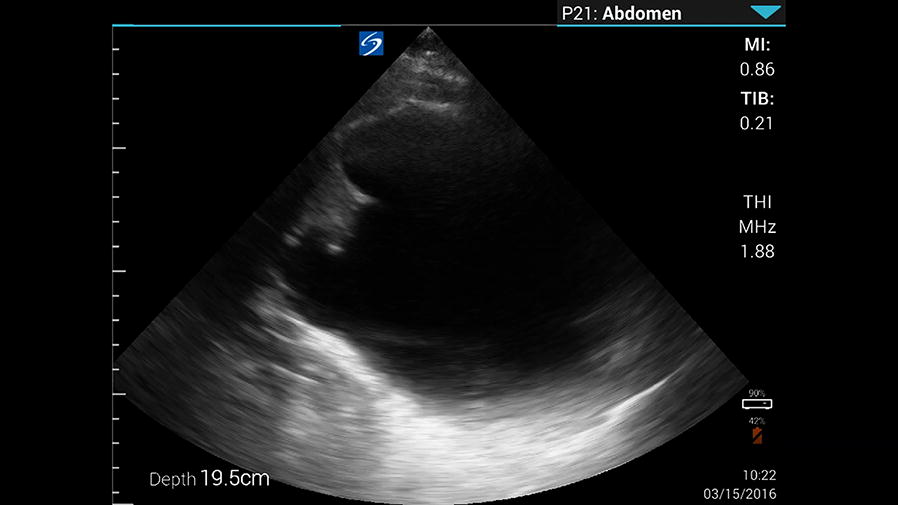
Ultrasound of the patient’s right upper quadrant. There is a large anechoic cystic structure with adjacent smaller cystic structures; these smaller cystic structures are actually the dilated biliary tree. These could easily be confused for daughter cysts that are commonly seen with hydatid cysts of the liver
